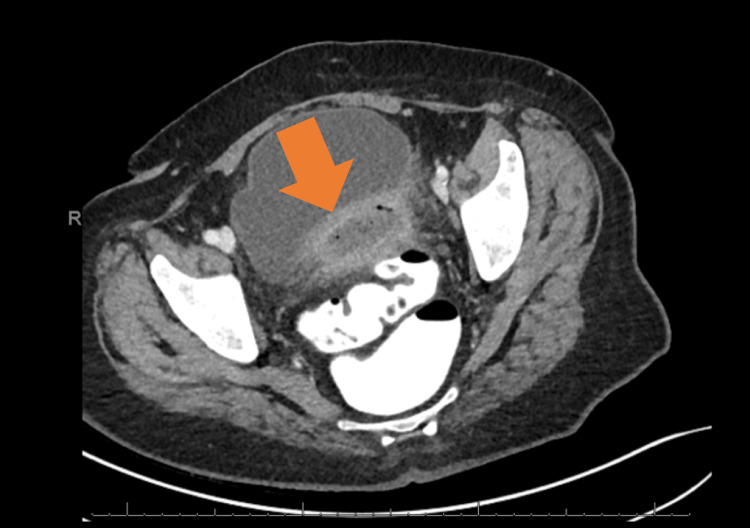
Preoperative CT scan demonstrating thick-walled, mildly enhancing complex fluid collection anterior to the sigmoid colon consistent with a perisigmoid abscess of diverticular origin. CT: computed tomography
